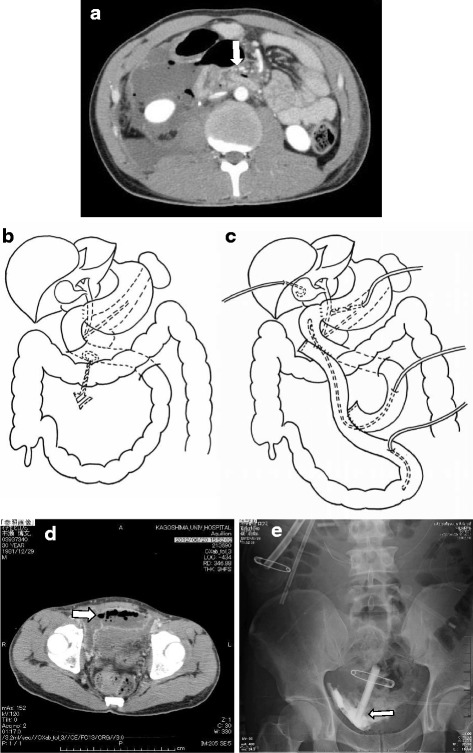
a CT scan showing rupture of the horizontal duodenum, free air, and perirenal fluid collection. b Perforation in the horizontal duodenum. c Side-to-side duodenojejunostomy was performed with decompressive gastrostomy and duodenostomy, feeding jejunostomy, and percutaneous transhepatic bile drainage. d Collection in the pelvic cavity 1 month post-surgery. e Plain radiogram showing abscess collection and percutaneous drainage tube in pelvic cavity
